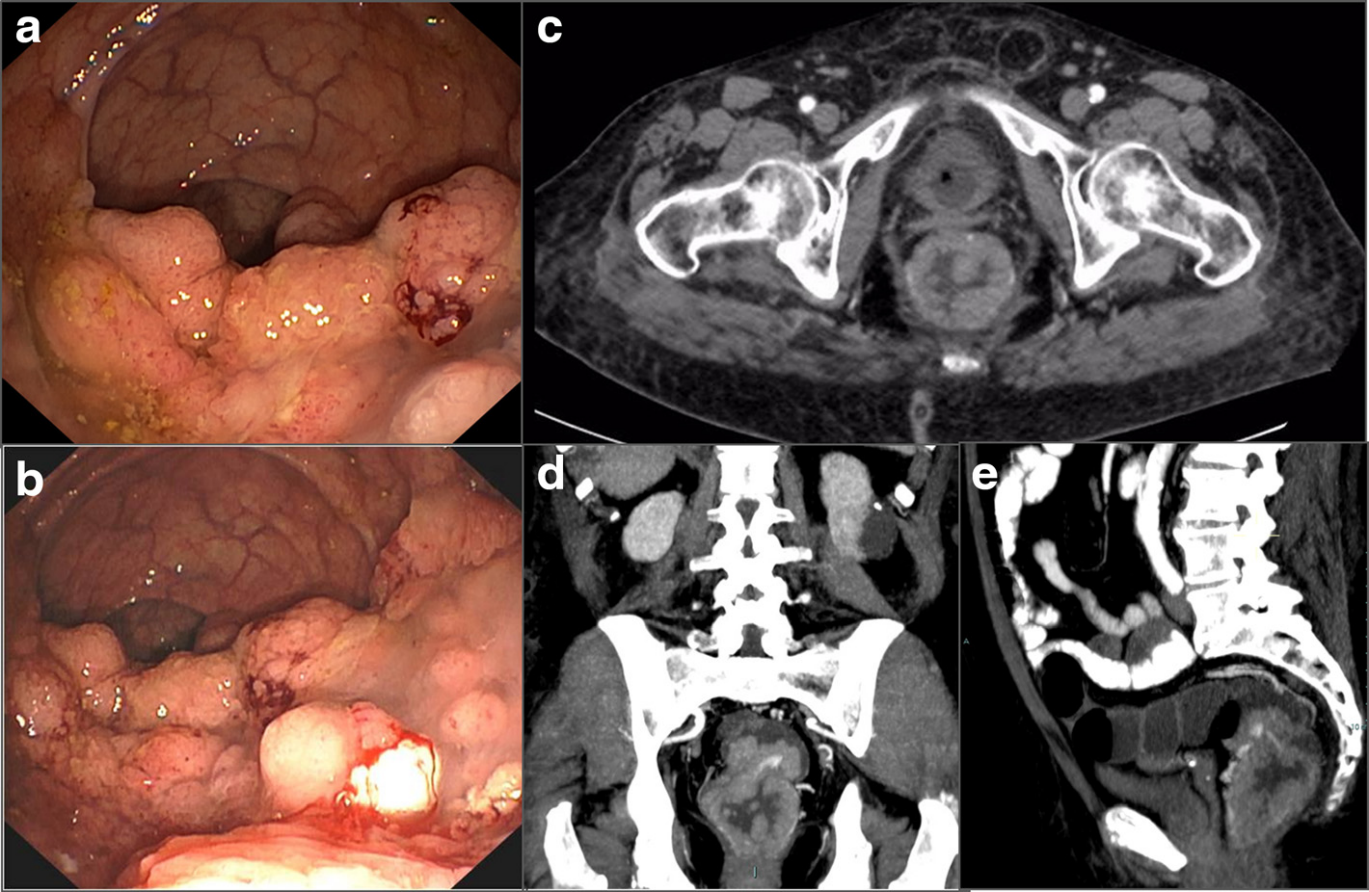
Diagnostic features of the giant rectal polyp. Panel a-b Colonoscopy revealing a large endoluminal polypoid mass involving the rectum; Panel c abdominal computed tomography revealing the presence of a giant villous tumor occupying the rectal lumen, transversal/axial view; Panel d coronal view; Panel e sagittal view
